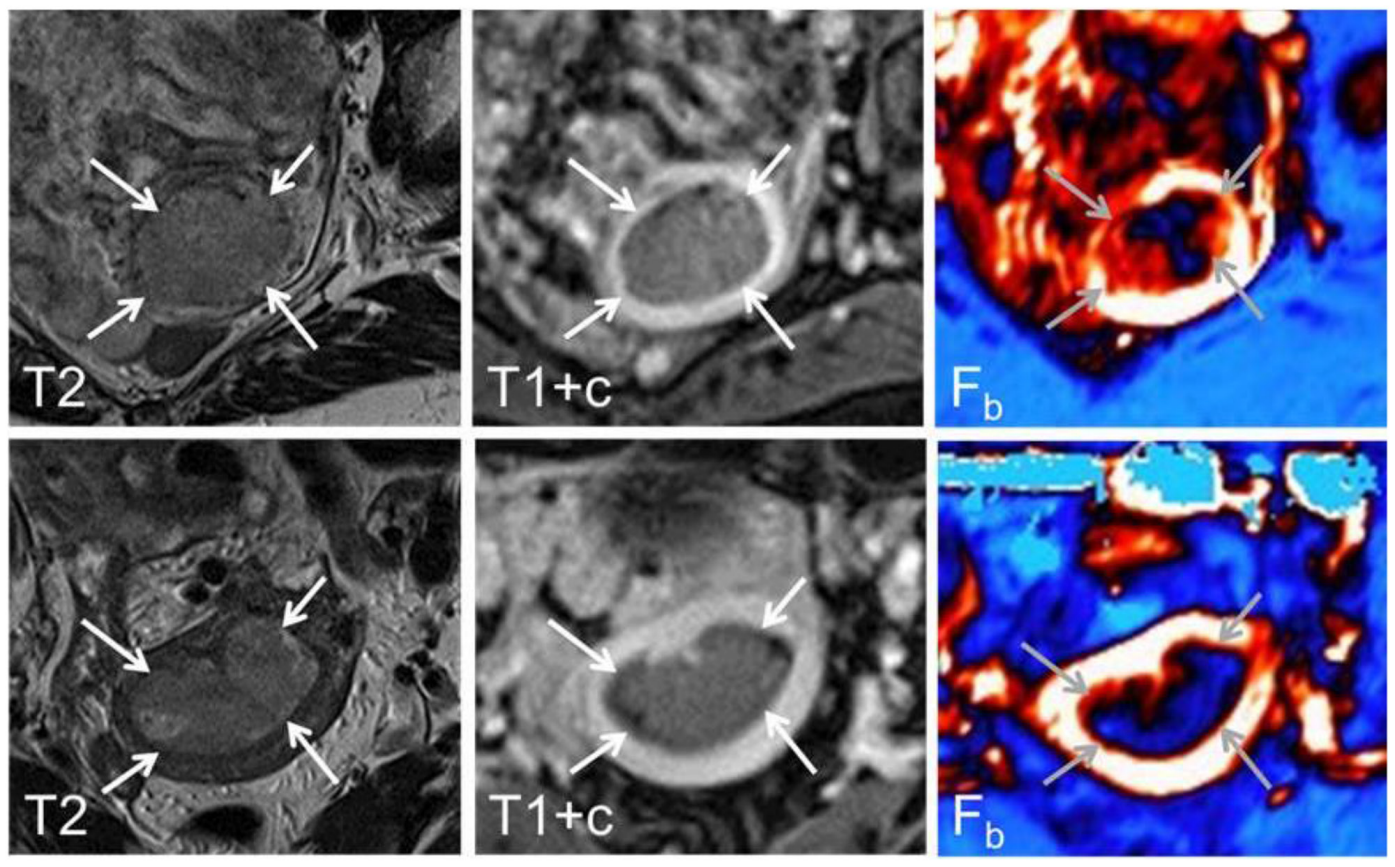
Axial oblique T2-weighted MRI (left panel), contrast-enhanced T1-weighted MRI (middle panel) and parametric blood flow (F_b_, right panel) map from a 68 year-old patient with FIGO stage 1B, grade 3 and high tumour F_b_ (upper panel) and a 52 year-old patient with FIGO stage 1B, grade 1 and low tumour F_b_ (lower panel). At conventional diagnostic MRI the endometrial carcinomas (arrows) are typically depicted as hyper-intense relative to the surrounding myometrium on T2-weigthed image while hypo-intense on contrast-enhanced T1-weighed image. On the corresponding parametric maps high blood flow is depicted as white/red while low blood flow is depicted as dark blue.
